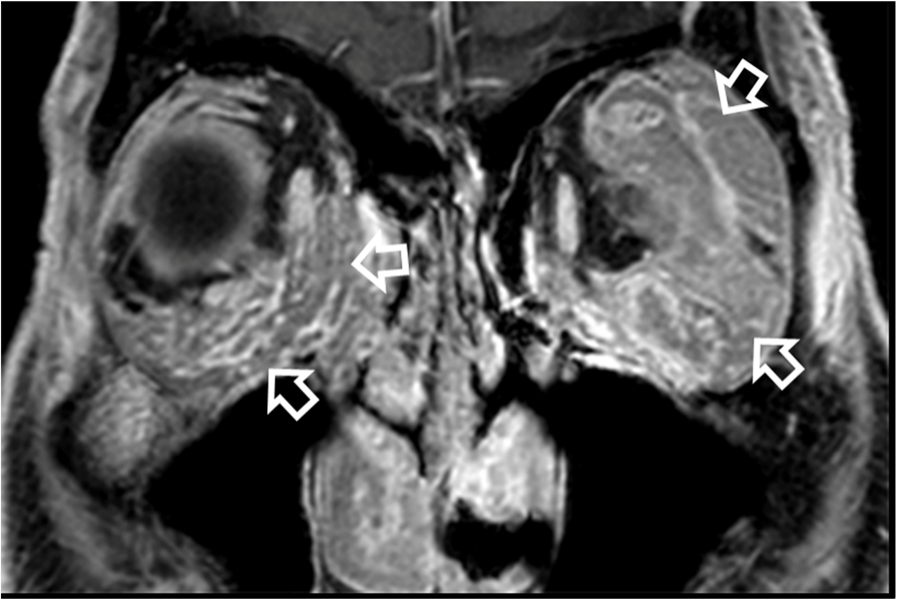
Magnetic resonance imaging of a 72-year-old man with bilateral orbital MALToma presenting as orbital swelling. A coronal T1-weighted post-contrast image with fat saturation reveals extensive bilateral extraconal and intraconal orbital involvement and involvement of the eyelids (open arrows)
